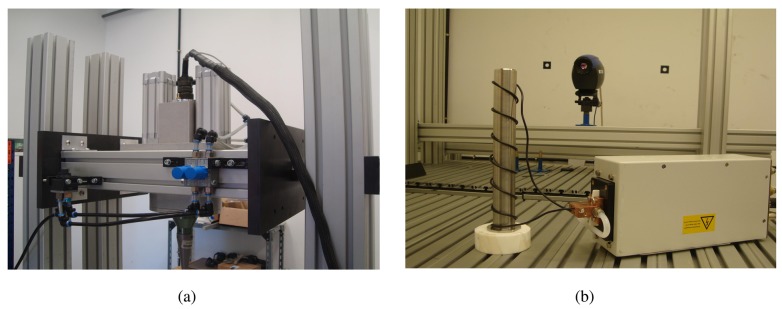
Ultrasound and induction stimulation techniques for NDT inspection using IRT. (a) Ultrasound gun. (b) Induction coil.
